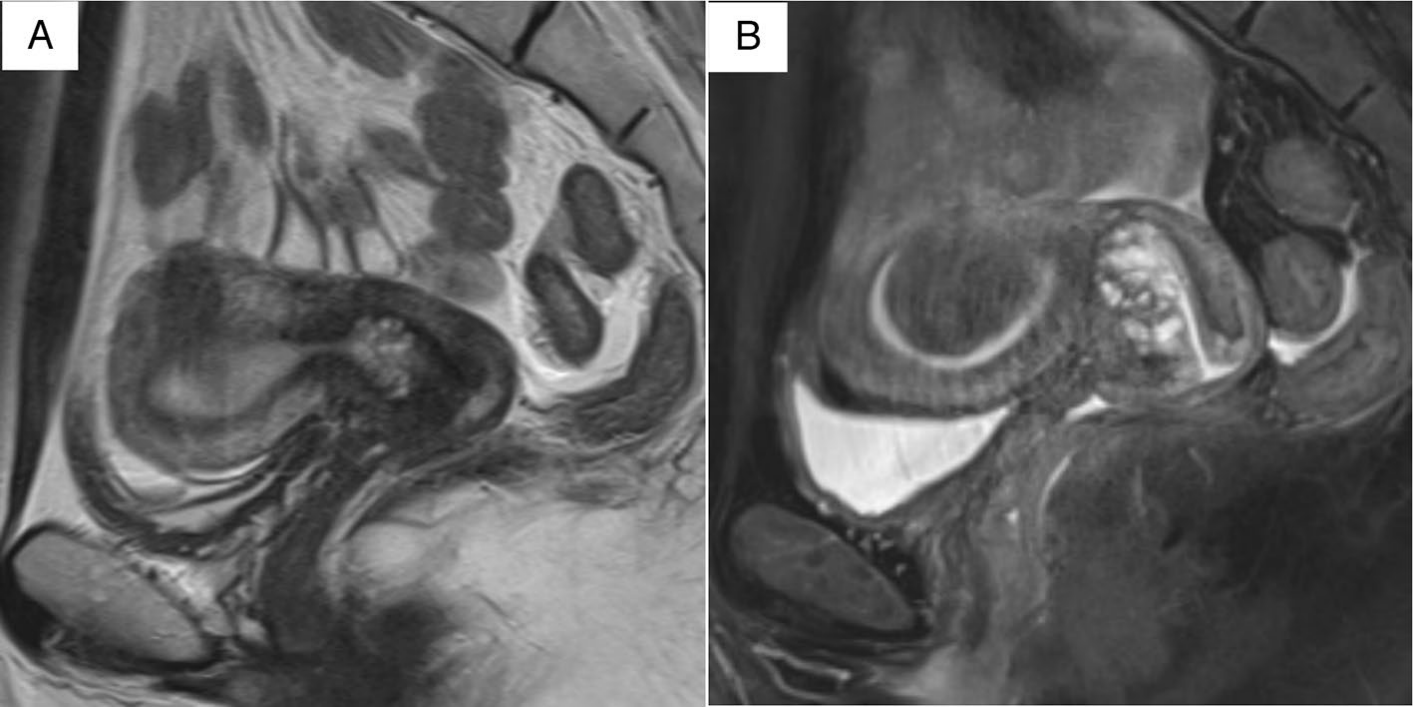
A Sagittal T2-weighted (T2WI) image of 34-year-old woman with lobular endocervical glandular hyperplasia shows tiny cysts in the upper part of the cervix. This lesion was classified as the “microcystic pattern.” B Sagittal fat-suppressed T2WI of the same patient at the age of 41 years shows that the lesion is composed of some small cysts (< 5 mm) and solid components in the central part of the lesion, surrounded by relatively large cysts (≥ 5 mm). This lesion was classified as the “typical cosmos pattern.” The lesion also increased in size
